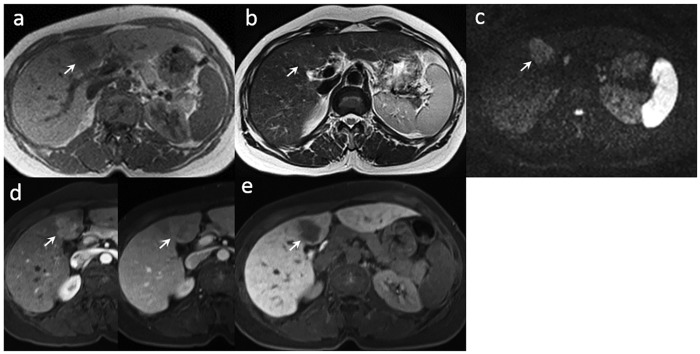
A 50-year-old female with moderately differentiated HCC (arrow) at S4 of the liver underwent gadoxetic acid-enhanced MRI and hepatectomy. (a) The tumor showed hypointensity on the T1-weighted image. (b) The tumor was isointense to the adjacent liver parenchyma on the T2-weighted image. (c) The tumor showed hyperintensity on diffusion-weighted imaging. (d) Hypervascularity was found in the tumor in the atrial phase, but was isointense to the adjacent liver parenchyma in the venous phase of dynamic MRI study. (e) The tumor was hypointense on the Gd-EOB-DTPA-enhanced hepatobiliary phase T1-weighted image.
